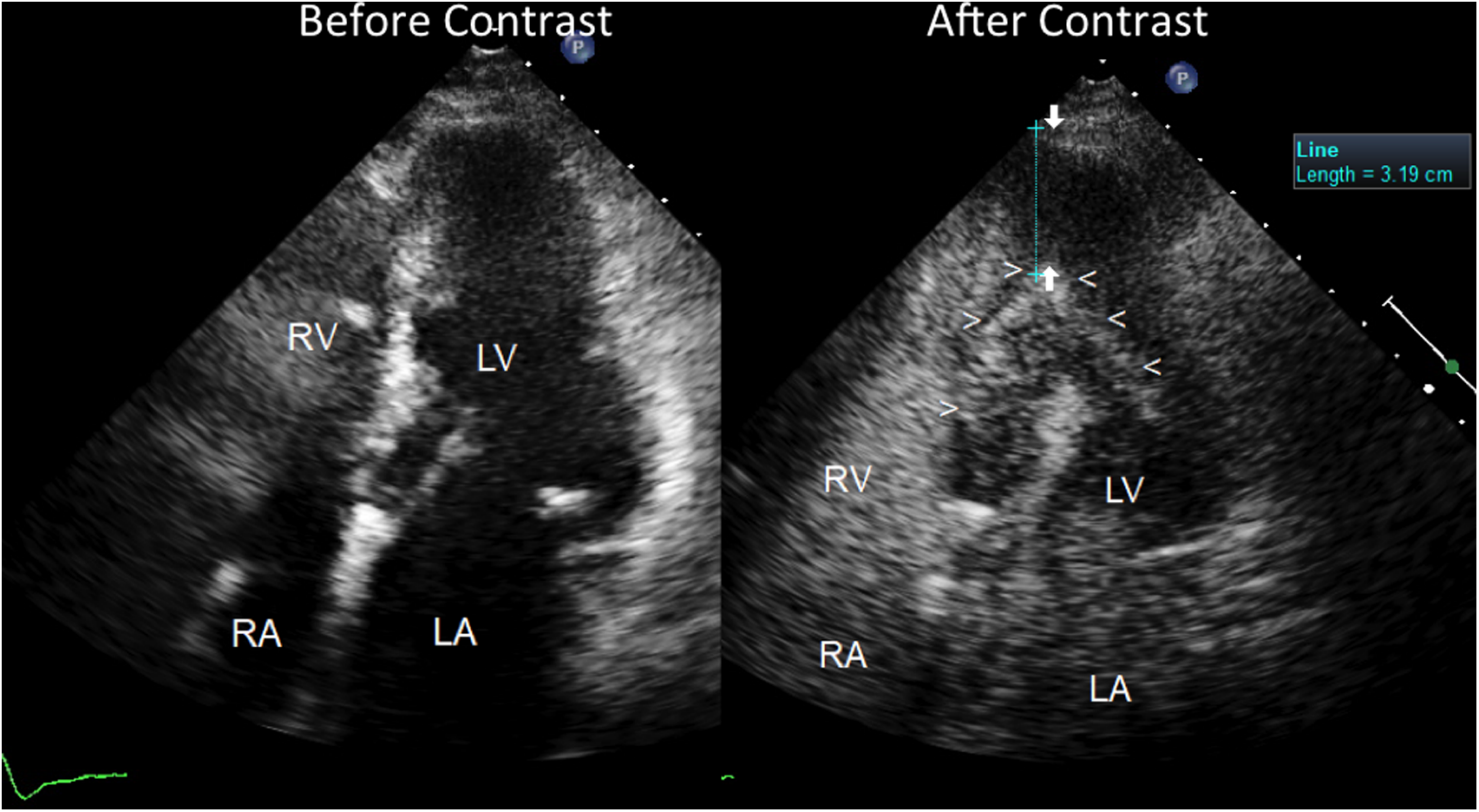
Severe apical left ventricular hypertrophy, as assessed by contrast enhanced transthoracic echocardiography. Four chamber view before (on the left) and after ultrasound contrast injection (on the right). Before contrast, the LV apical region does not show any clear delineation of the endocardial border but reveals a fairly homogeneous low backscatter echo-structure resembling a blood pool. No evident abnormality of the LV apical region can be noted but just poor quality imaging of the apical region with scarce delineation of the endocardium; the pace-maker leads can be noted in the right atrial cavity. On the contrary, after contrast injection (Sonoview® 1 cc in bolus) (on the right), a perfect delineation of the true apical endocardium is very clearly depicted as a hyper reflective line of backscatter (arrowheads). The severe hypertrophy of the LV apical wall can be noted and measured, as indicated by arrows (>3 cm thickness). The left atrium is dilated. The right atrial cavities are filled with contrast medium. LV = left ventricle; RV = right ventricle; RA = right atrium; LA = left atrium.
